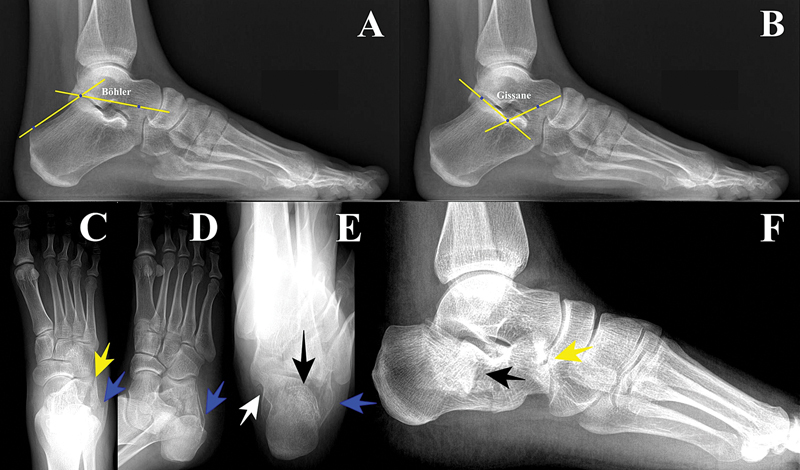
Radiographic evaluation. (
